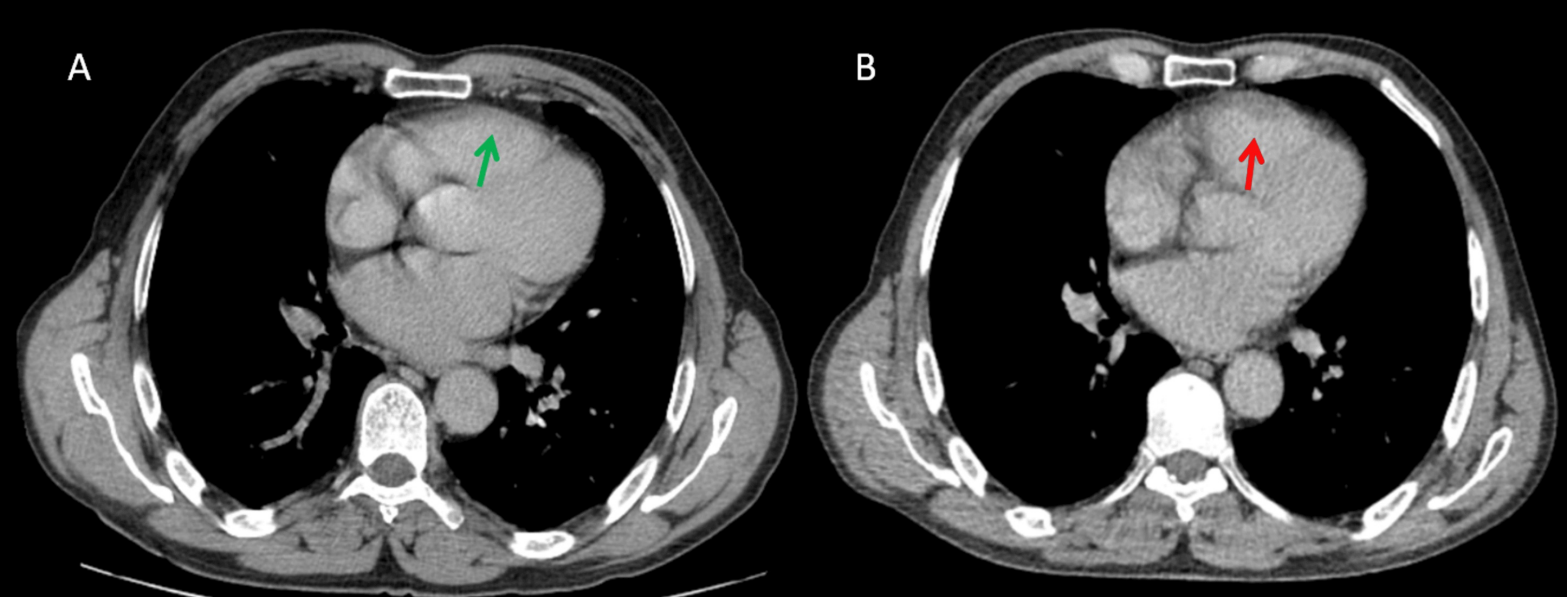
Axial CT scan slices at the level of the cardiac chambers. (A) One-year post-surgical follow-up CT scan demonstrating no cardiac abnormalities, with a preserved right ventricular wall (green arrow). (B) Two-year follow-up CT scan showing an ill-defined hypodense tissue thickening of the right ventricular wall, raising suspicion of a pathological process but remaining non-specific (red arrow).
